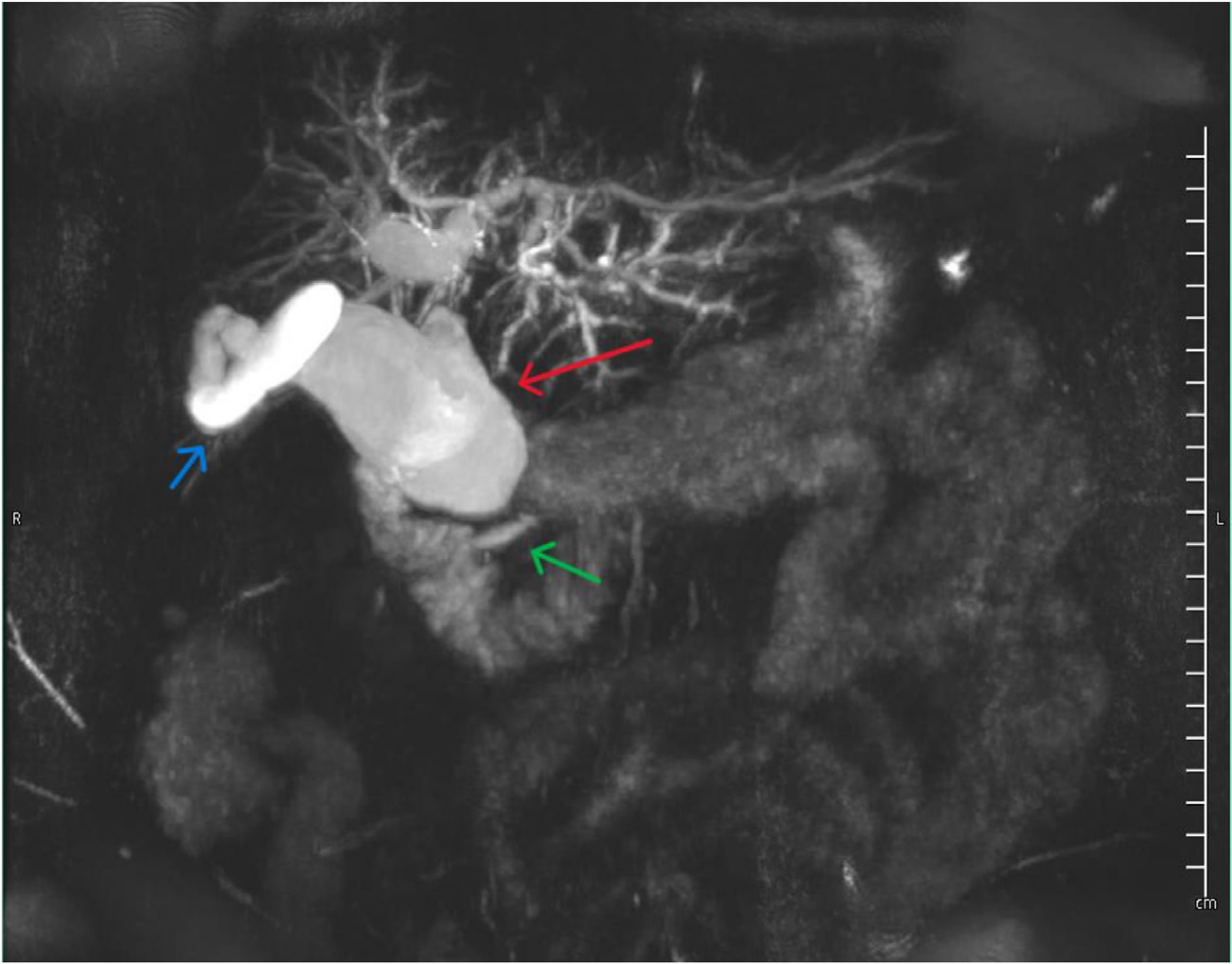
Abdominal MRI/ MRCP coronal Maximum Intensity Projection reconstructed image shows a long common channel (green arrow) suggestive of PBM. Significant dilation of the common bile duct (red arrow) is noted into which the gallbladder and prominent cystic duct drain (blue arrow) consistent with Type 1A choledochal cyst.
